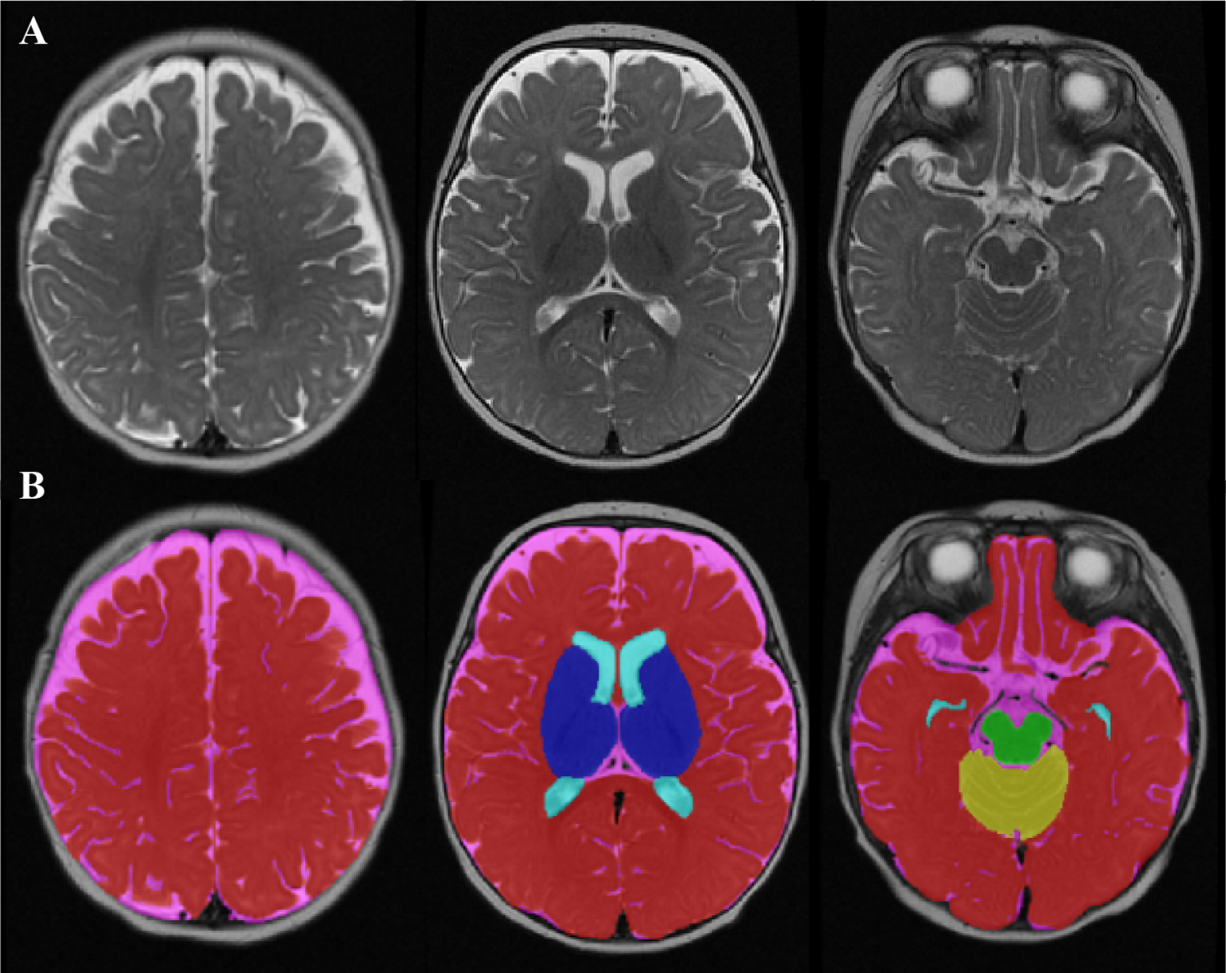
Volumetric segmentation of a 4-month-old brain. a T2-weighted axial MRI image of a 4-month-old infant brain. b The final result of the volumetric segmentation, with label maps for CSF (*pink*), lateral ventricles (*light blue*), midbrain (*green*), cerebellum (*yellow*), subcortical grey matter (*dark blue*), and total grey and white matter (*red*)
